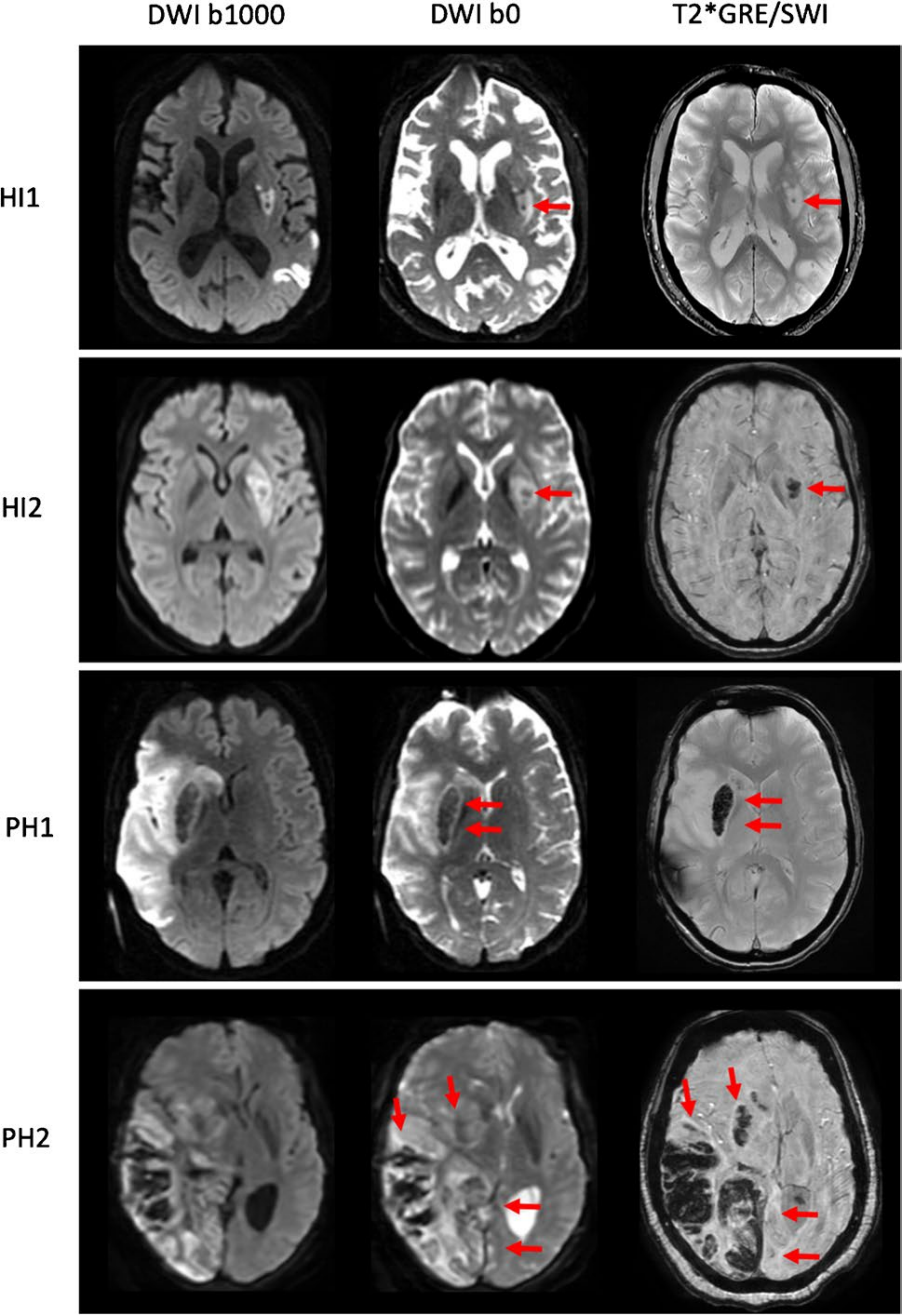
Detection of intracranial hemorrhage (ICH) on diffusion-weighted imaging (DWI) b0 and T2*GRE/SWI. Axial slices of four examinations are displayed from top to bottom showing HI1, HI2, PH1, and PH2 types of ICH indicated by the red arrows
